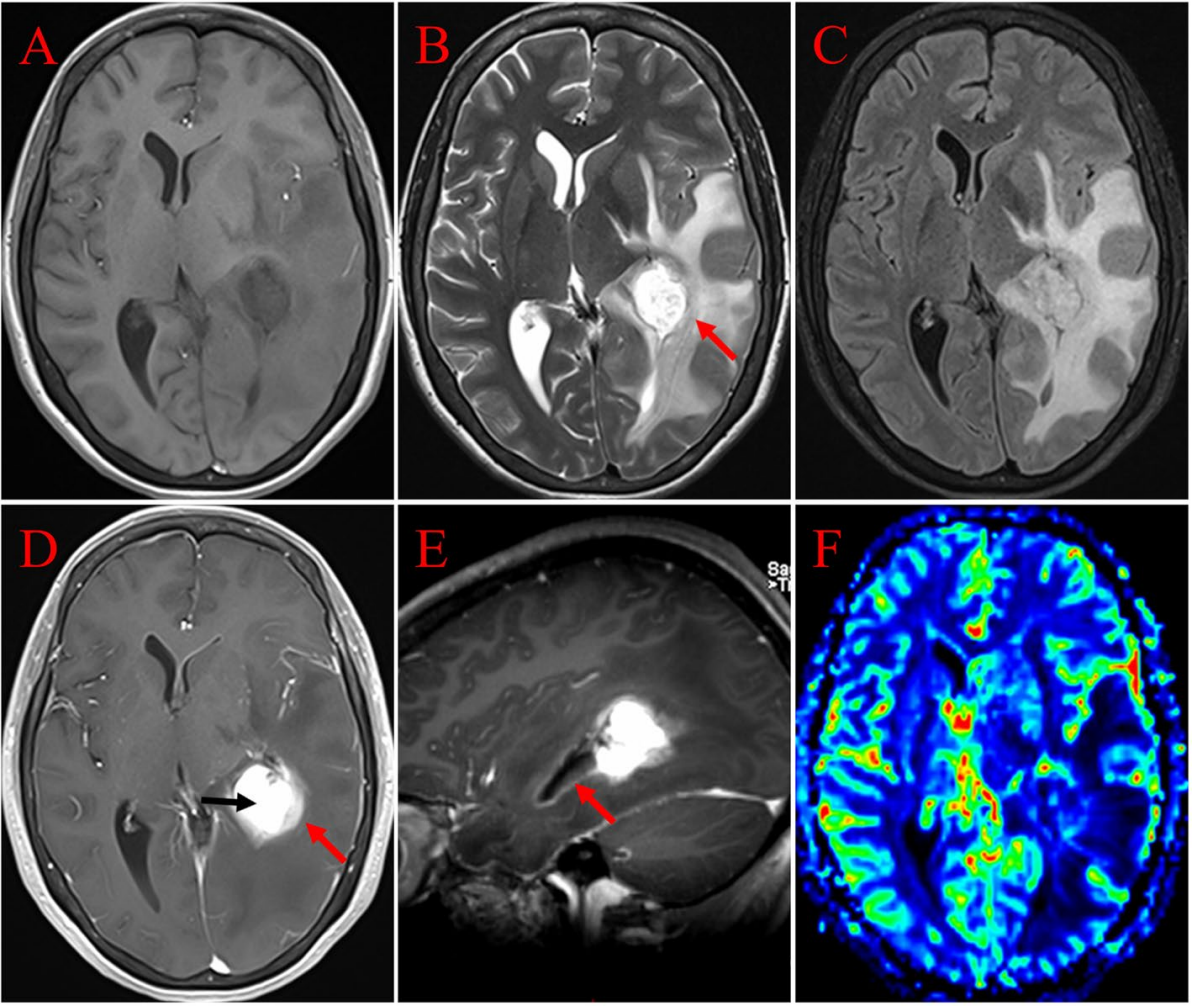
MRI shows a well-circumscribed, lobulated mass in the left lateral ventricle trigone, with marked perilesional brain edema. A On the axial T1WI, the lesion displays low to intermediate signal intensity compared to that of the gray matter. B On the axial T2WI, the tumor displays heterogeneous high signal intensity with a few hypointense areas, and a low signal ring surrounding the neoplasm (arrows). C On the axial FLAIR, the lesion shows heterogeneous hyperintensity. D On the axial contrast-enhanced MRI, the tumor demonstrates significant enhancement and appears with the halo sign, the distinctive halo (red arrows) shows relatively weak enhancement compare to that of the mass (black arrows). E On the sagittal contrast-enhanced MRI, the lateral ventricular wall showed enhancement (red arrows). F On the corresponding color CBV image, the tumor shows decreased perfusion
